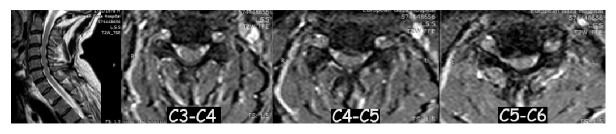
Preoperative cervical magnetic resonance image (MRI) demonstrated multilevel cervical disc with myelopathy C3-C4, C4-C5, C5-C6.
